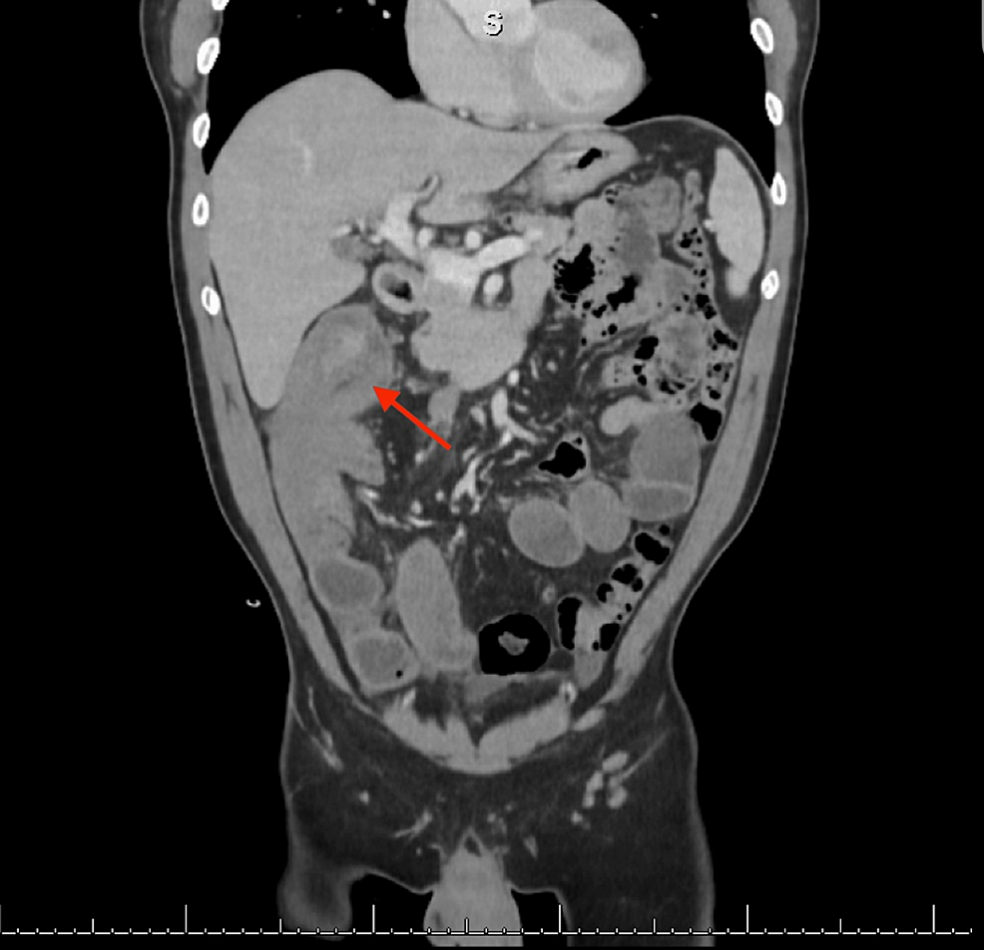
CT of the abdomen and pelvis with intravenous contrast, sagittal view.
